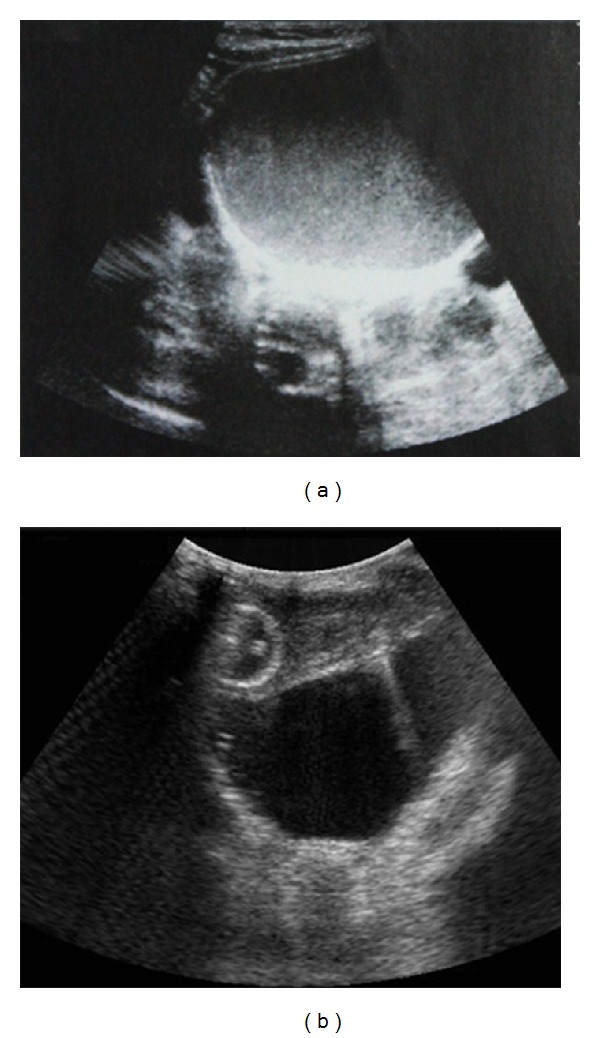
Pelvic US. (a) Transverse view shows a large well-defined cystic mass with internal echoes, which could easily be misinterpreted as overly distended urinary bladder. Note the bilateral ureteral dilatation. (b) Insertion of a Foley catheter makes it clear that the cystic mass is separate from the urinary bladder which is severely compressed and therefore hard to detect on ultrasound.
